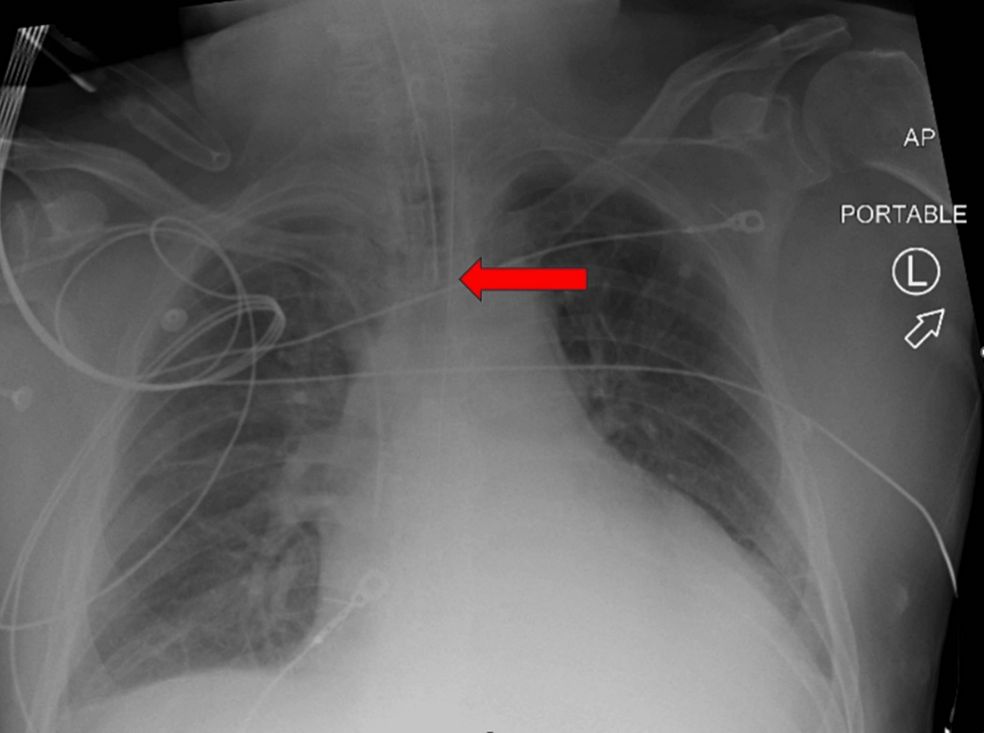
Chest X-ray upon arrival at the tertiary center (red arrow highlighting the end of endotracheal tube)
